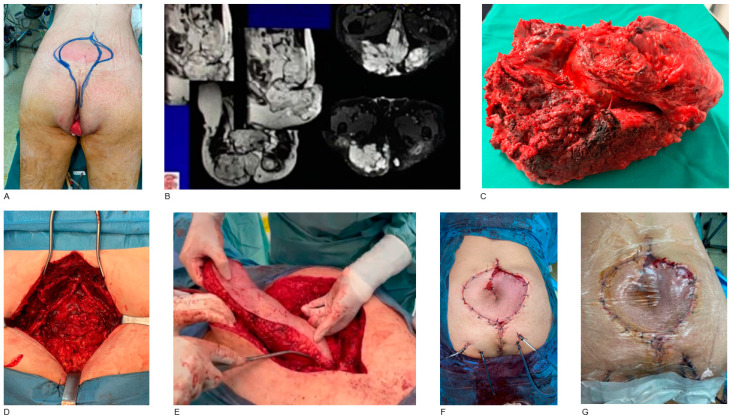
Sacral chordoma resection and sacrum reconstruction with VRAM flap. A 58-year-old male patient with a sacral chordoma. Pre-operative marking of the wide-field resection margins (A). T2-weighted and T2-weighted fat sat MR images of the sacrum with intravenous contrast. Sacral chordoma is demonstrated as a hyperintense lesion with irregular margins (B). En-block resection of tumor and sacrum (C). The patient is in prone position. Tissue and volume defect was created after sacral chordoma wide-field resection. The small intestine is visible, as the defect is connected with the peritoneal cavity (D). Transfer of VRAM flap from the abdominal wall, through the peritoneal cavity and pelvis, to the sacral area (E). Post-operative result after sacrum reconstruction with transpelvic VRAM flap (F). Application of negative pressure wound therapy (NPWT) aiming at a proper wound healing process and avoidance of wound dehiscence (G).
